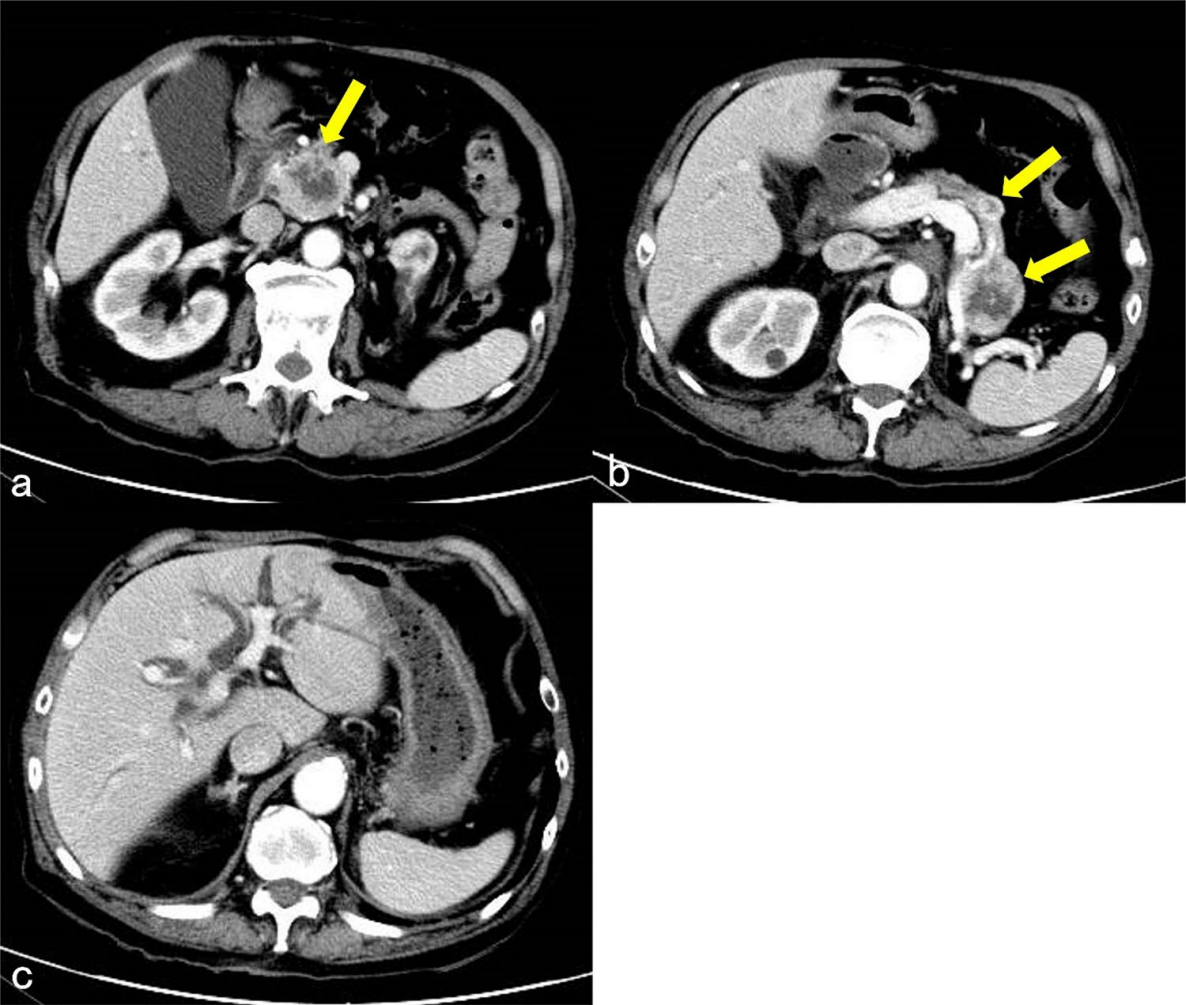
a, b Contrast-enhanced computed tomography showed multiple tumors in the pancreas (arrow). c The intrahepatic bile duct was dilated
